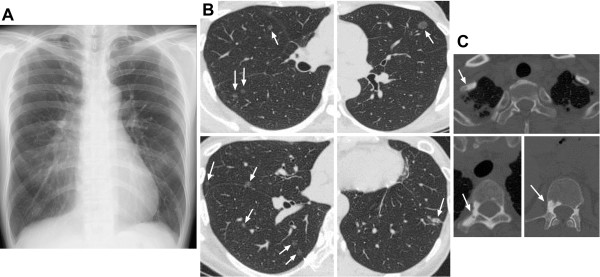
Chest radiograph and computed tomography findings at our patient’s first visit.  (A) Chest radiograph demonstrating pleural wall thickening on the right side of the apex and upper lung field. (B) Multiple nodular lesions, typically 10mm or less in diameter, can be observed throughout the lung fields on high-resolution chest computed tomography scan (arrows). The nodular lesions are mostly ground-glass opacities, while some have a higher density. (C) A chest computed tomography scan demonstrating the bone sclerosis lesions at Th3, Th6, and the first costal bone (arrows).
